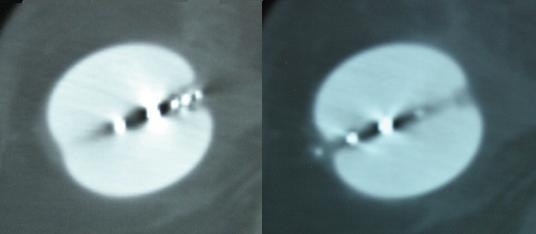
CT scan slices from case 2 showing the symmetry of the center channel.
